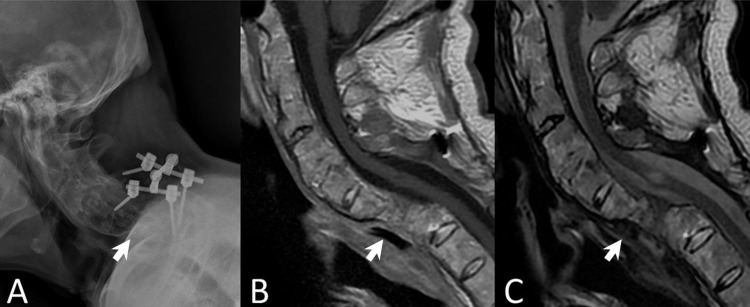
Disappearance of the cage A: Lateral radiograph taken at 22 months after the initial operation. The metallic cage was no longer appreciated. B: Sagittal T1-weighted magnetic resonance imaging (MRI) demonstrated no spinal cord compression. C: Sagittal T2-weighted MRI demonstrated no spinal cord compression.
